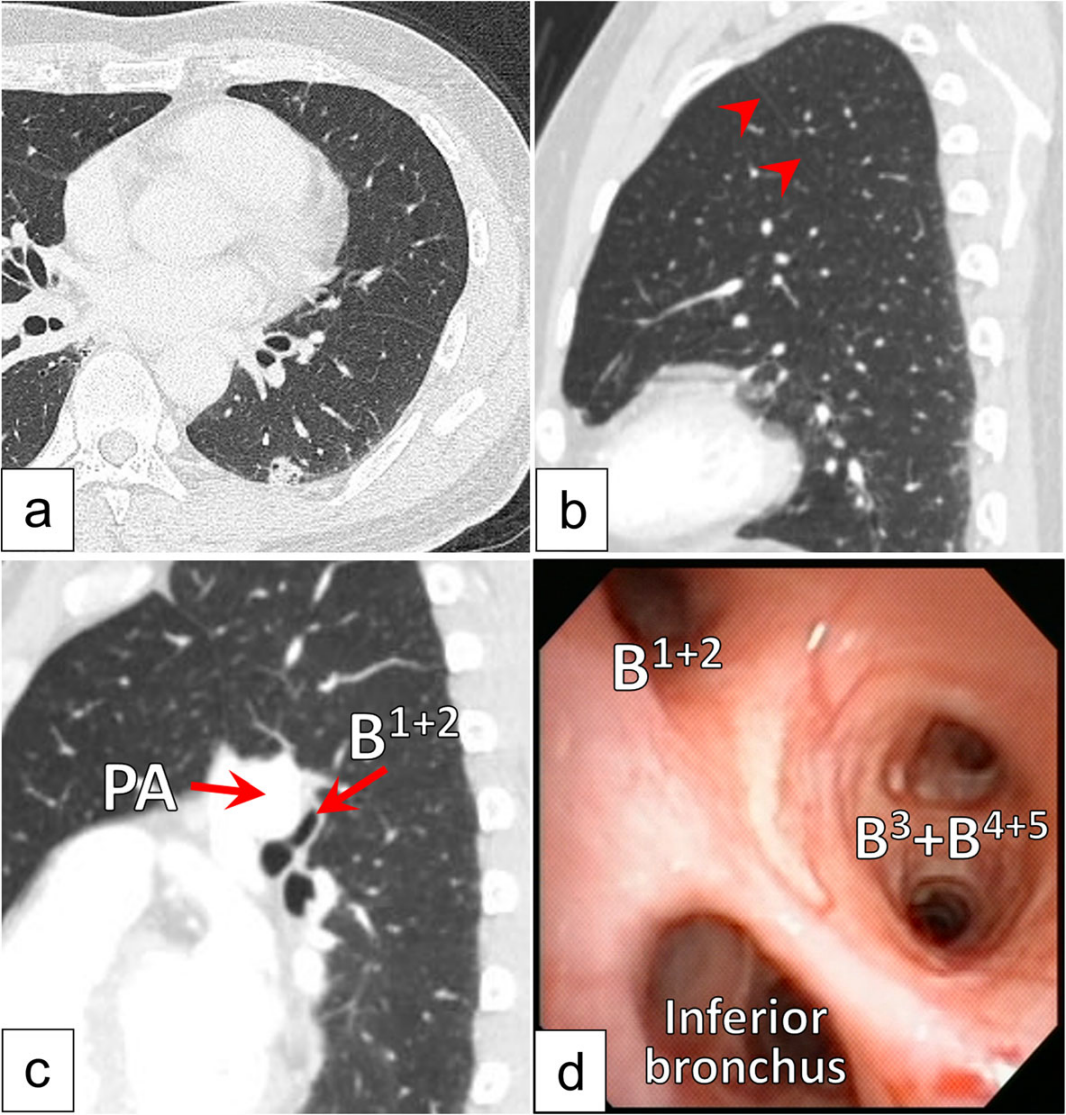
Preoperative chest computed tomography images and bronchoscopic image. Chest computed tomography (CT) revealed a tumor in the left inferior lobe (a) and hyperlobulation between S^1 + 2^ and S^3^ (red arrow), while the interlobar fissure between S^1 + 2^ and S^6^ was completely fused (b). CT revealed a displaced B^1 + 2^ running behind the main pulmonary artery (c). Bronchoscopy revealed that three bronchi branched from the left main bronchus (d).
